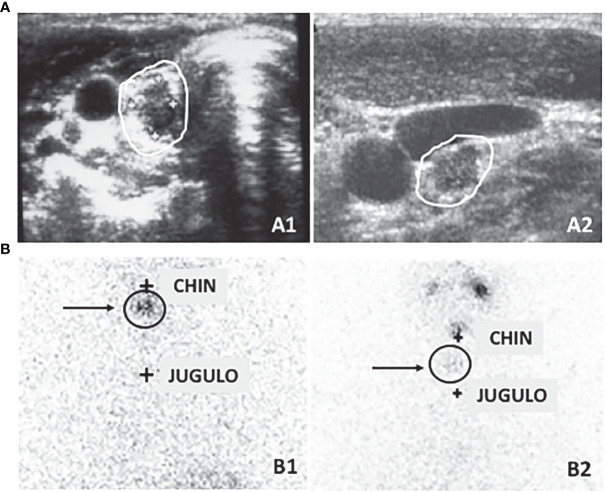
(A) Neck ultrasound can detect small lesions that cannot be clearly interpreted as normal or pathological lesions such as sub-centimetric thyroid bed lesion (A1) referable to either thyroid remnant tissue or cancer persistence and indeterminate small cervical lymph nodes (A2). (B) Similarly, thyroid bed scintigraphy can detect faint uptake in the thyroid bed that can be due to both a normal remnant or a local persistence or recurrence of the disease (B1) or a small lymph node (B2).
